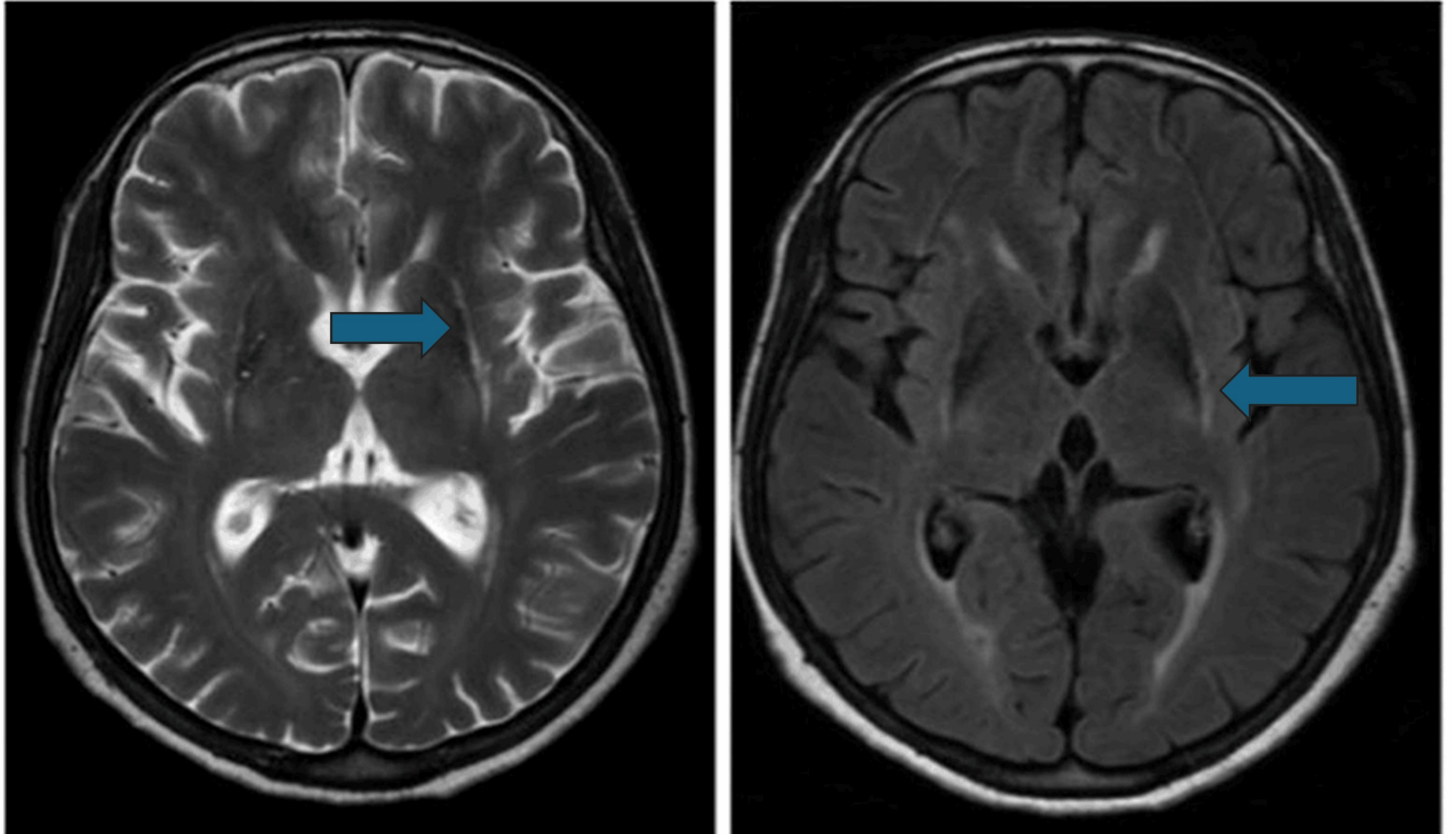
T2 and FLAIR axial images at the level of basal ganglia: a hyperintense rim along the lateral margin of the bilateral putamen (hyperintense putaminal rim sign) in MSA-P. Atrophy of the posterior aspect of the bilateral putamen is also noted. Arrows in the two images are pointing toward the hyperintensity of the bilateral putamen (putaminal rim sign). FLAIR = fluid-attenuated inversion recovery; MSA-P = multiple system atrophy-parkinsonian
